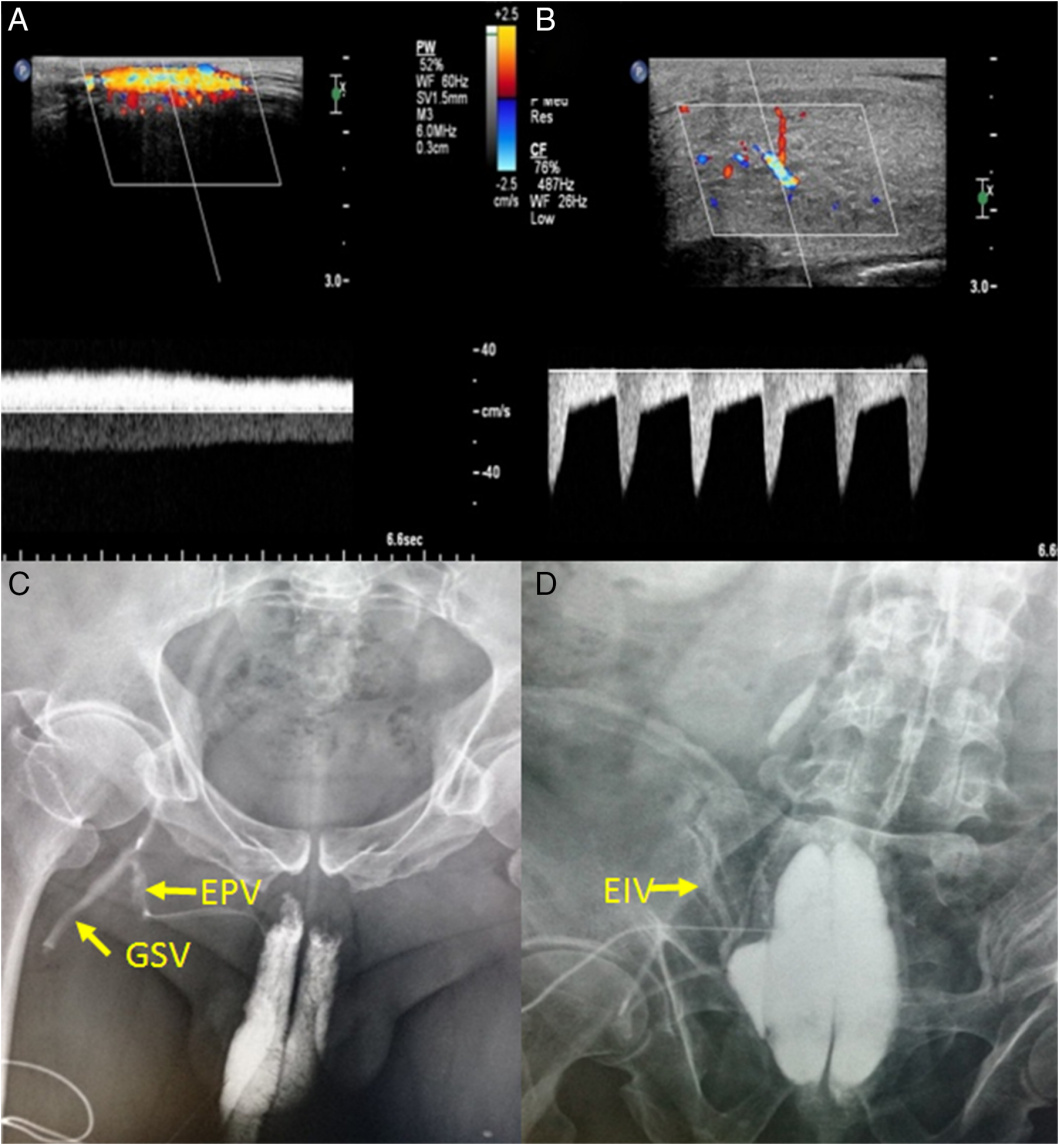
Cavernosal leakage of the penis in a 54‐year‐old‐man, mostly occurring in the external pudendal vein, who underwent a segmented ligation of the deep dorsal vein 2 years ago. A and B, The ultrasonographic images showed continuous blood flow signal in the superficial dorsal vein but no signal in the deep dorsal vein. The spectrum of the cavernous artery showed normal PSV, EDV > 7 cm/s, and RI < 0.80. He was finally diagnosed with superficial dorsal venous leak by ultrasound. C and D, The cavernosography showed contrast enhancement of the external pudendal vein, the great saphenous vein, and the external iliac vein on the right side. EDV indicates end‐diastolic velocity; EIV, external iliac vein; EPV, external pudendal vein; GSV, great saphenous vein; PSV, peak systolic velocity; RI, resistance index.
